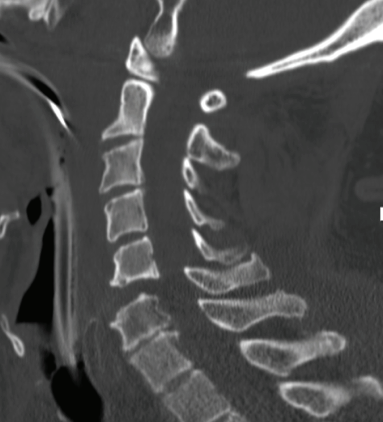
Sagittal cervical spine CT reconstruction-image at our institution shows dramatically worsened subluxation at C5-C6.
